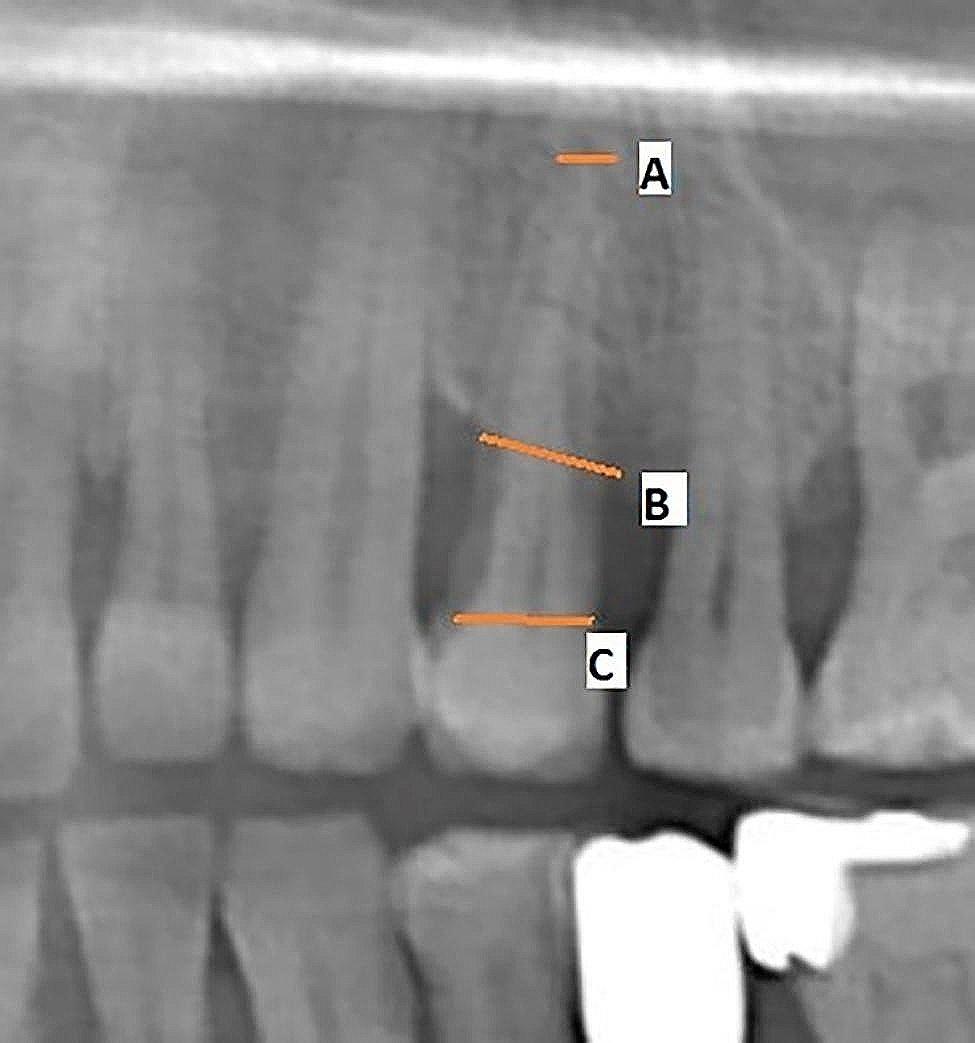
Measuring bone loss on a panoramic radiograph. A: apex of the root, B: bone level, C: cemento-enamel junction. AC/CB shows the rate of bone loss
